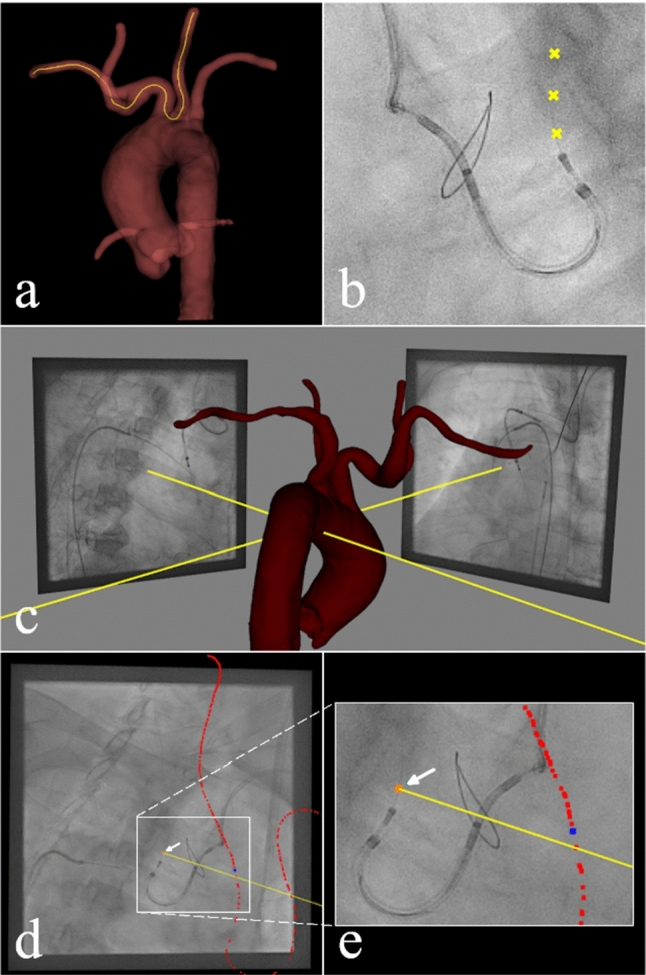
General method: (a) Extraction of centreline (orange line) of right subclavian artery and left common carotid artery from the aortic model generated from the preprocedural CT using 3DSlicer. (b) Example cut-out of placed markers (yellow) along the guidewire in the left common carotid artery in an x-ray projection. (c) Co-registration of preprocedural data and peri-procedural x-ray system based on two monoplane projections of the aortic arch with different angulations. (d) 3D localization example of one 2D marker (orange, arrow): determination the point (blue point) of the centreline (red points) whose projection line is most parallel to the projection line (yellow line) of the 2D marker. (e) Close-up of the white box in (d) for better visualization
